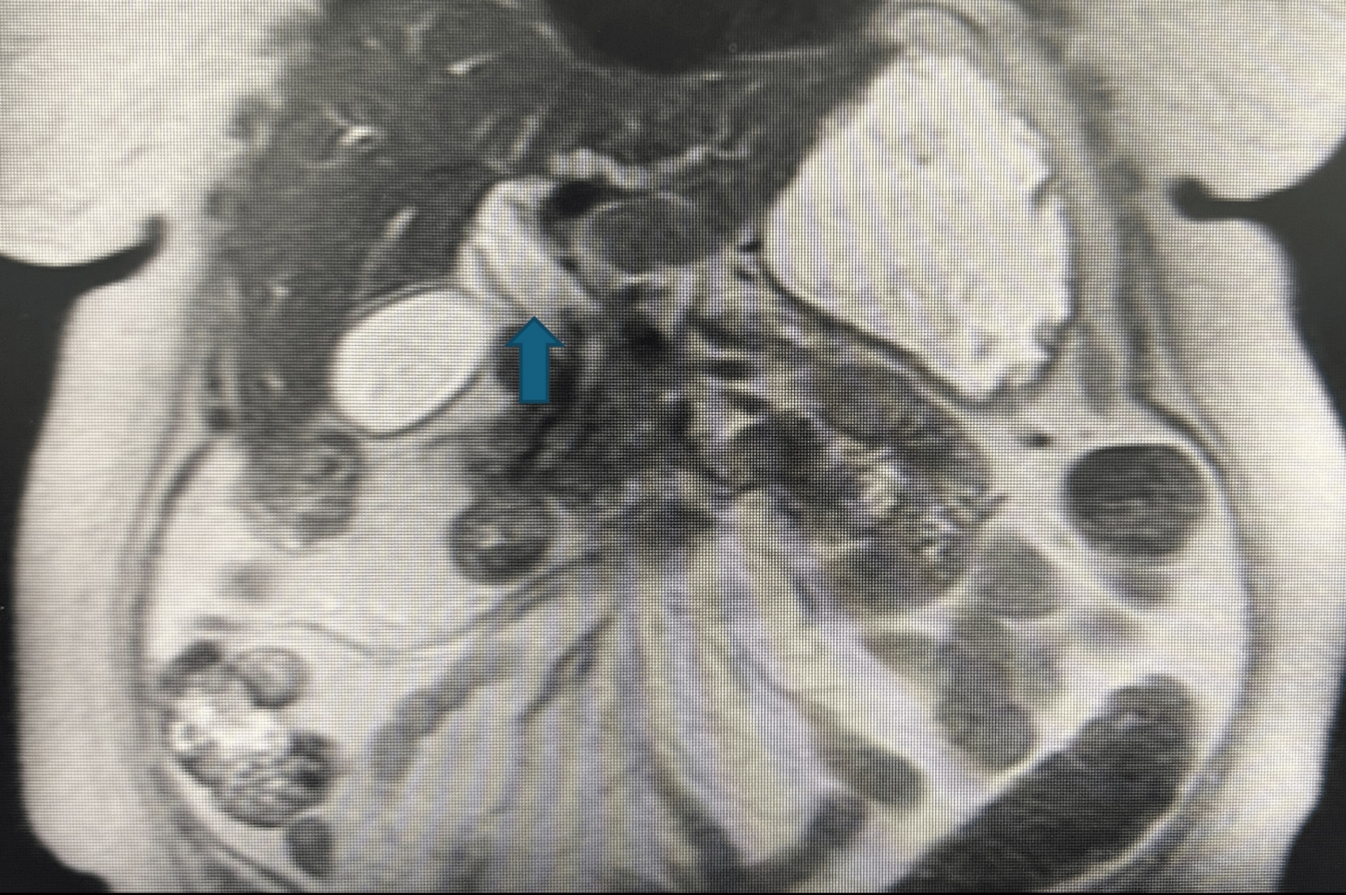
Magnetic resonance image showing dilated biliary ducts.
